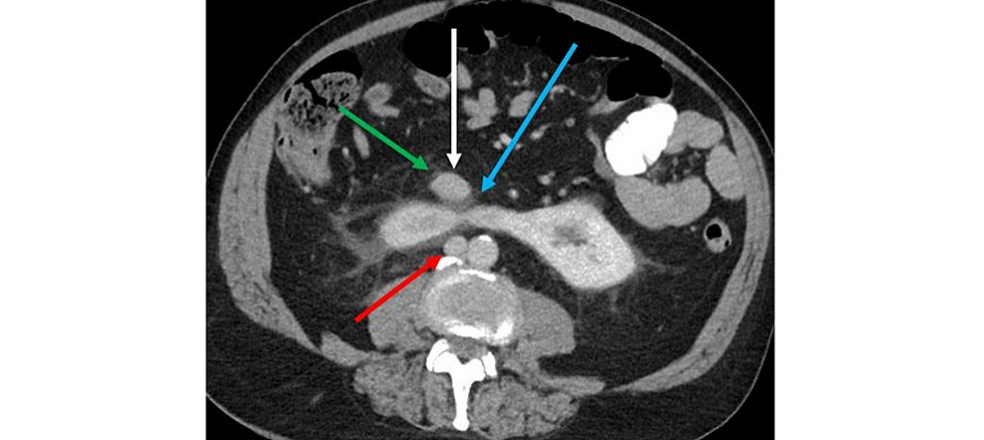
Axial contrast-enhanced CT with intravenous and oral contrast demonstrates a horseshoe kidney with a pre-isthmic IVC (white arrow) and a posterior IVC (red arrow). Right gonadal vein (green arrow) drains into the pre-isthmic IVC. Narrowed right retrocaval ureter (blue arrow) between the pre-isthmic IVC and the horseshoe kidney. IVC: inferior vena cava
